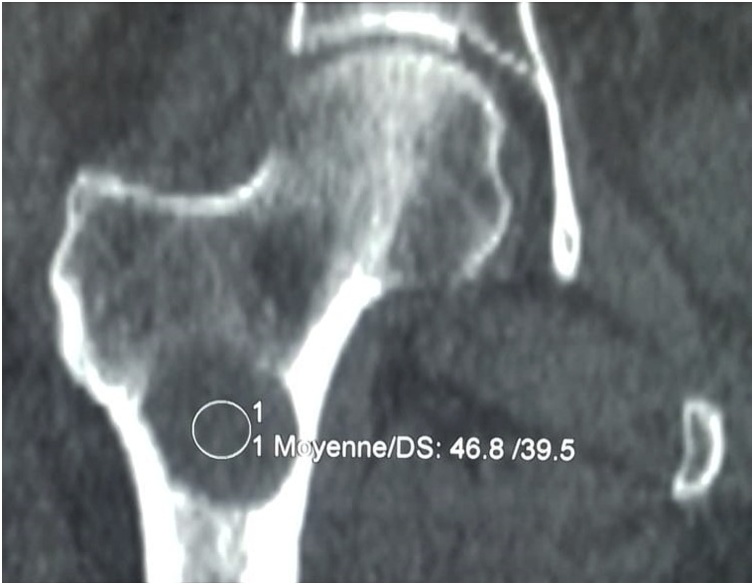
CT image of a lytic bone lesion of the upper femoral metaphysis.
